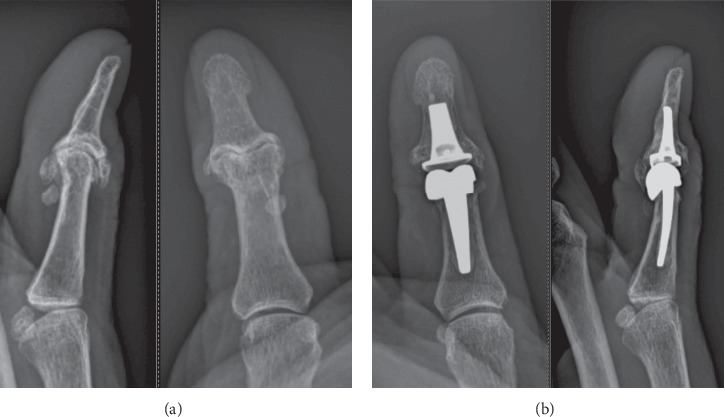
Pre- and postoperative X-rays.
